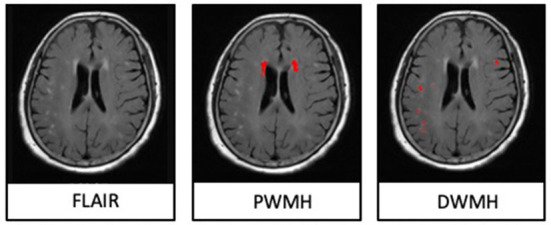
A representative case with shown final periventricular white matter hyperintensities (PWMHs) and deep white matter hyperintensities (DWMHs) segmentations on FLAIR.
